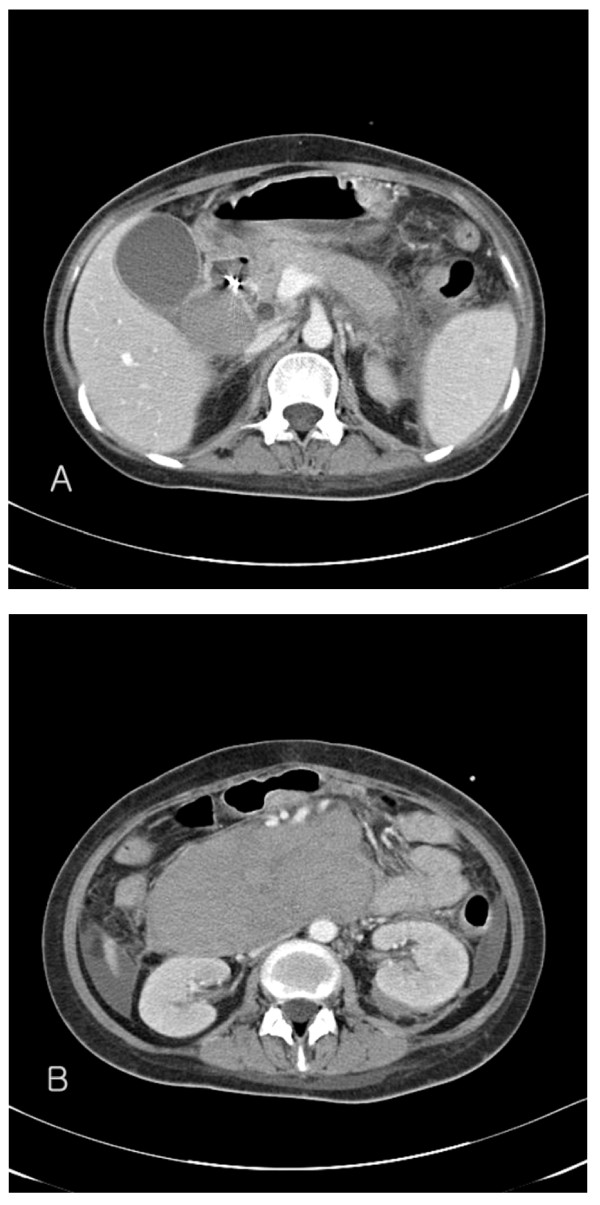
CT of the abdomen demonstrates a mass with a filling defect in the second portion of the duodenum and marked narrowing of the lumen of the third portion of the duodenum (A), and the largest part of the hematoma in diameter (B).
